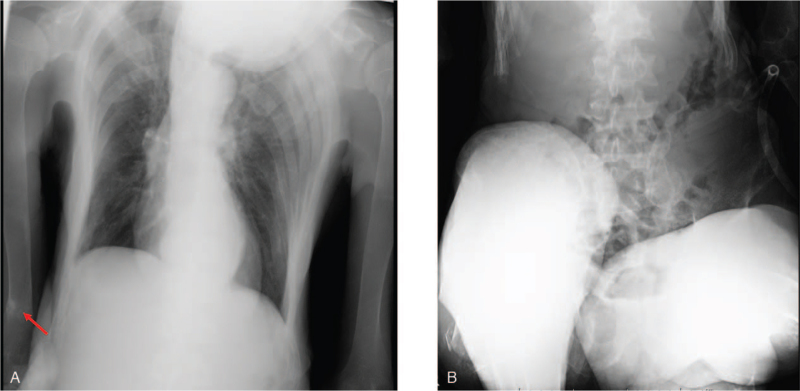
(A) In radiograph, there was looser's zone in humerus (a red arrow) which indicates low mineralization area and is characteristic for osteomalacia. (B) There was severe deformity in the long canal bone.
